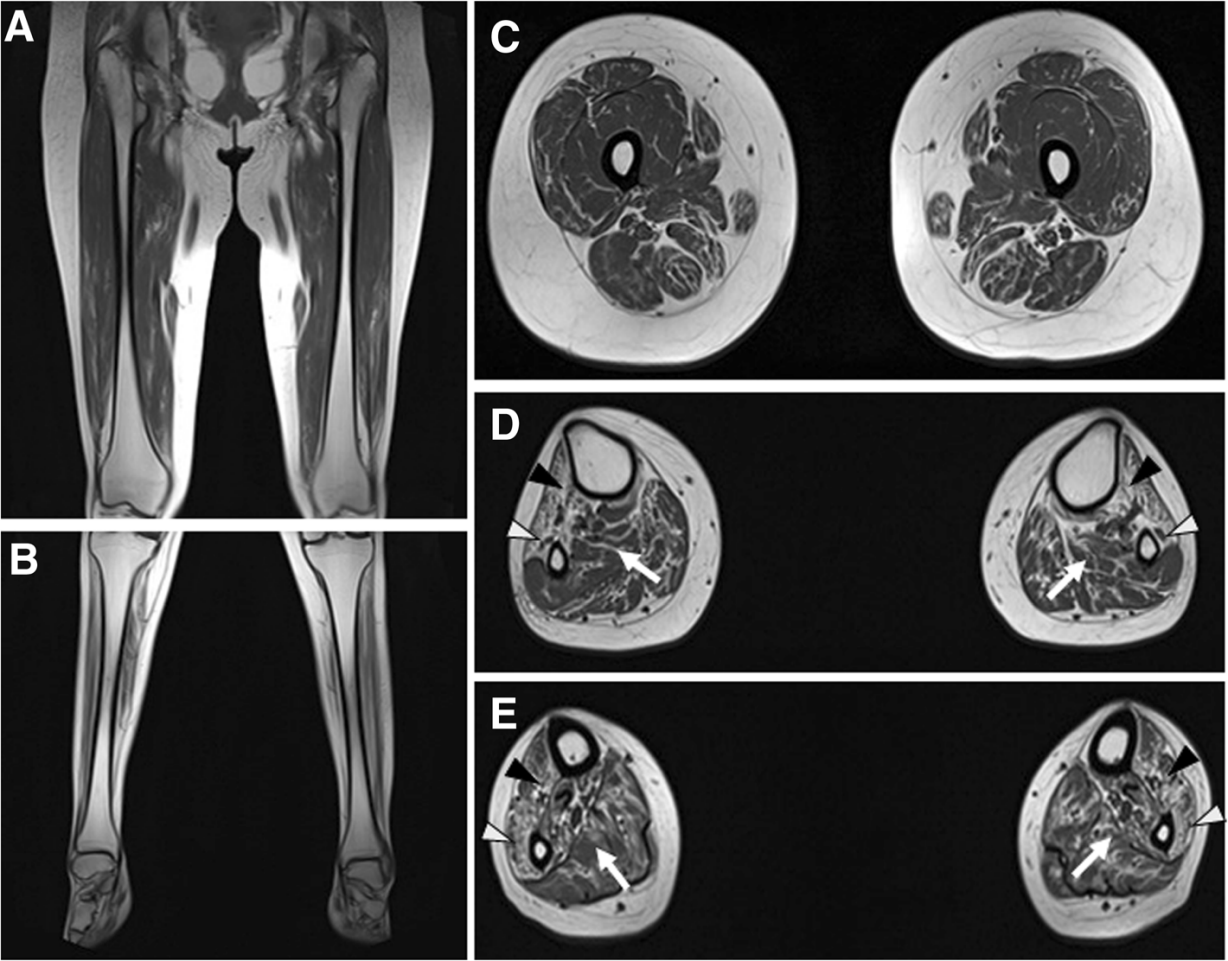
T1-weighted MRI of the proband (III-1). (A, B) Coronal images of thigh (A) and lower leg (B). T1-weighted coronal leg MRIs of lower extremities demonstrated more severe fatty replacement and muscle atrophies in the lower leg than did those of the thigh. (C) Axial image of the middle thigh. Vastus lateralis, semimembranous muscles showed moderate to severe fatty replacements, but they revealed more muscles than fat. However, sartorius, gracilis, biceps femoris, and rectus femoris muscles revealed fatty streaks. (D, E) Axial images at the upper third (D) and lower third calf (E). T1-weighted images revealed predominantly severe involvement of anterior (black arrowhead; anterior tibialis, extensor hallucis longus and digitorum longus) and lateral (white arrowhead; peroneus longus and brevis) compartment muscles, and superficial and deep posterior compartment (arrow; soleus and tibialis posterior) muscles showed mild involvement.
